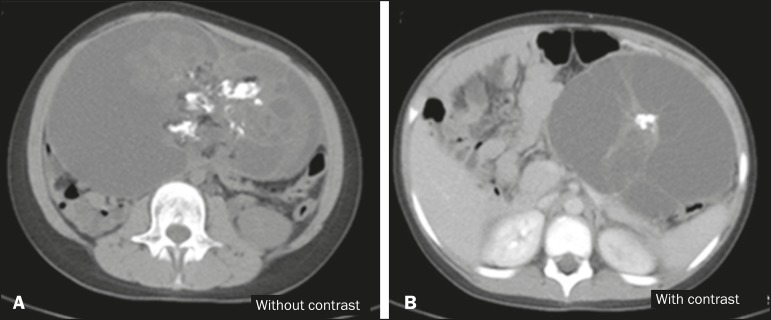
A: Non-contrast-enhanced axial CT scan showing an extensive solid-cystic
